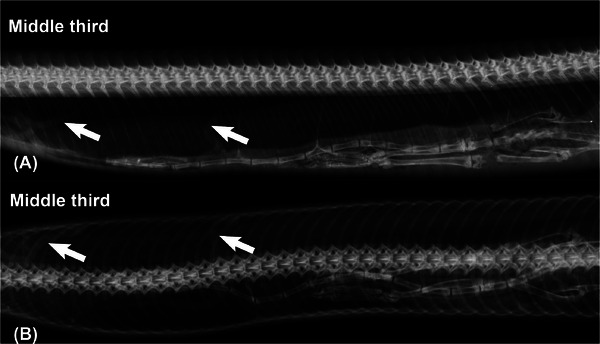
Lateral (A, middle third) and dorsoventral (B, middle third) radiograph of the coelomic cavity of a Southern American bushmaster (*Lachesis rhombeata*). Cylindrical structures in the lung fields are (arrows) compatible with pentastomids. After necropsy, pentastomid infection by *Porocephalus stilesi* was confirmed.
